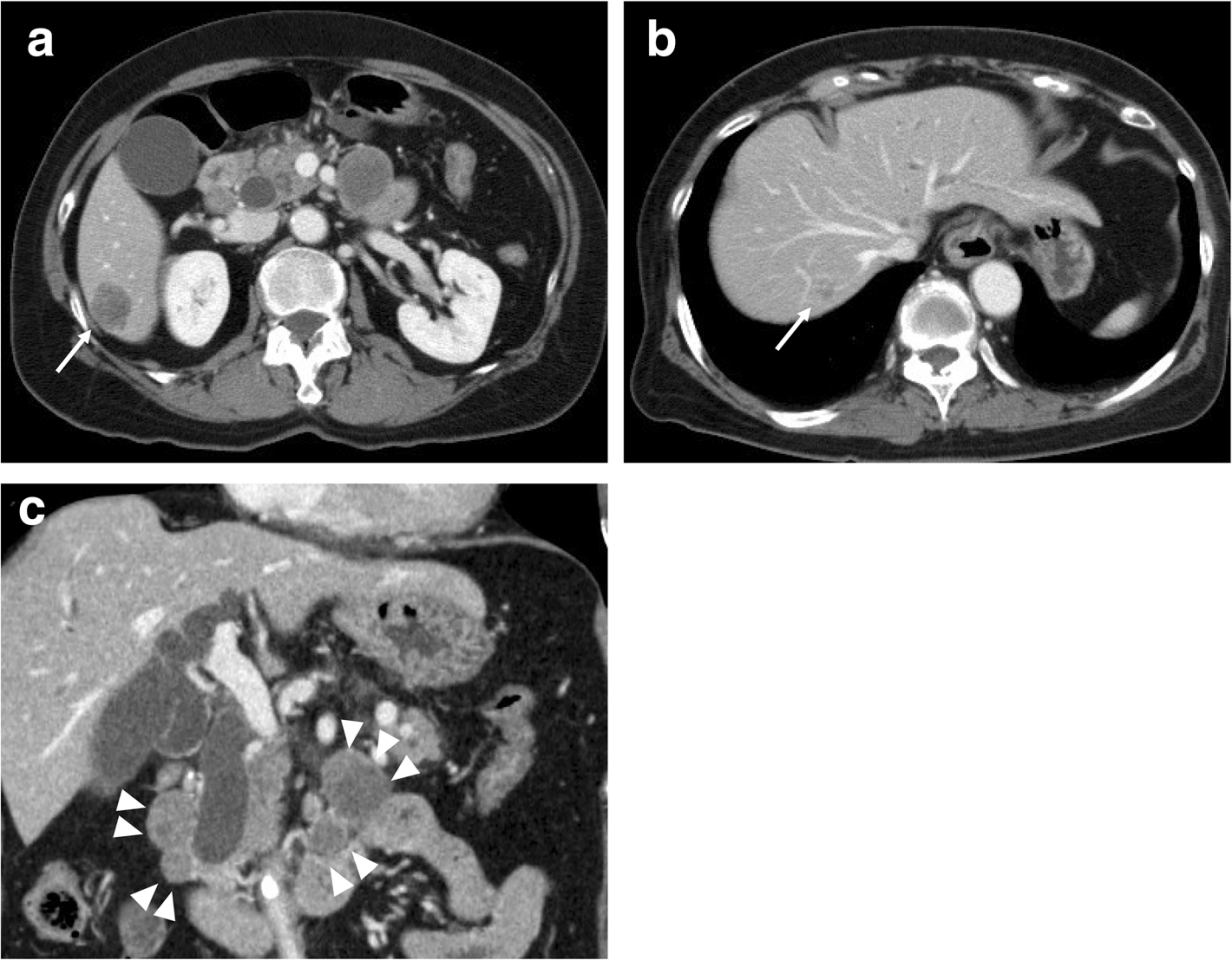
a, b Abdominal computed tomography showed dilatation of the intrahepatic and extrahepatic bile duct. Two hypovascular lesions were detected in the liver (arrow). c Multiple enlarged lymph nodes were present around the distal bile duct and superior mesenteric artery (arrowhead)
